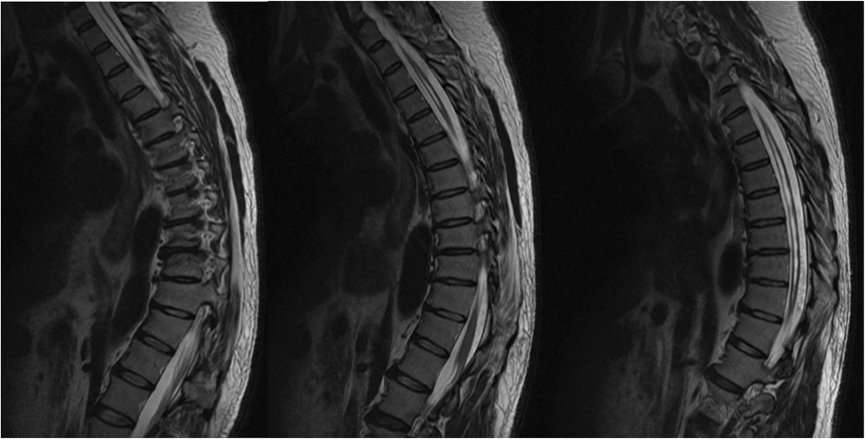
Magnetic resonance imaging of the thoracic spine. Sequential T2 fast recovery fast spin echo sequence sagittal cuts demonstrating the syrinx extending inferiorly down to T11 level.
